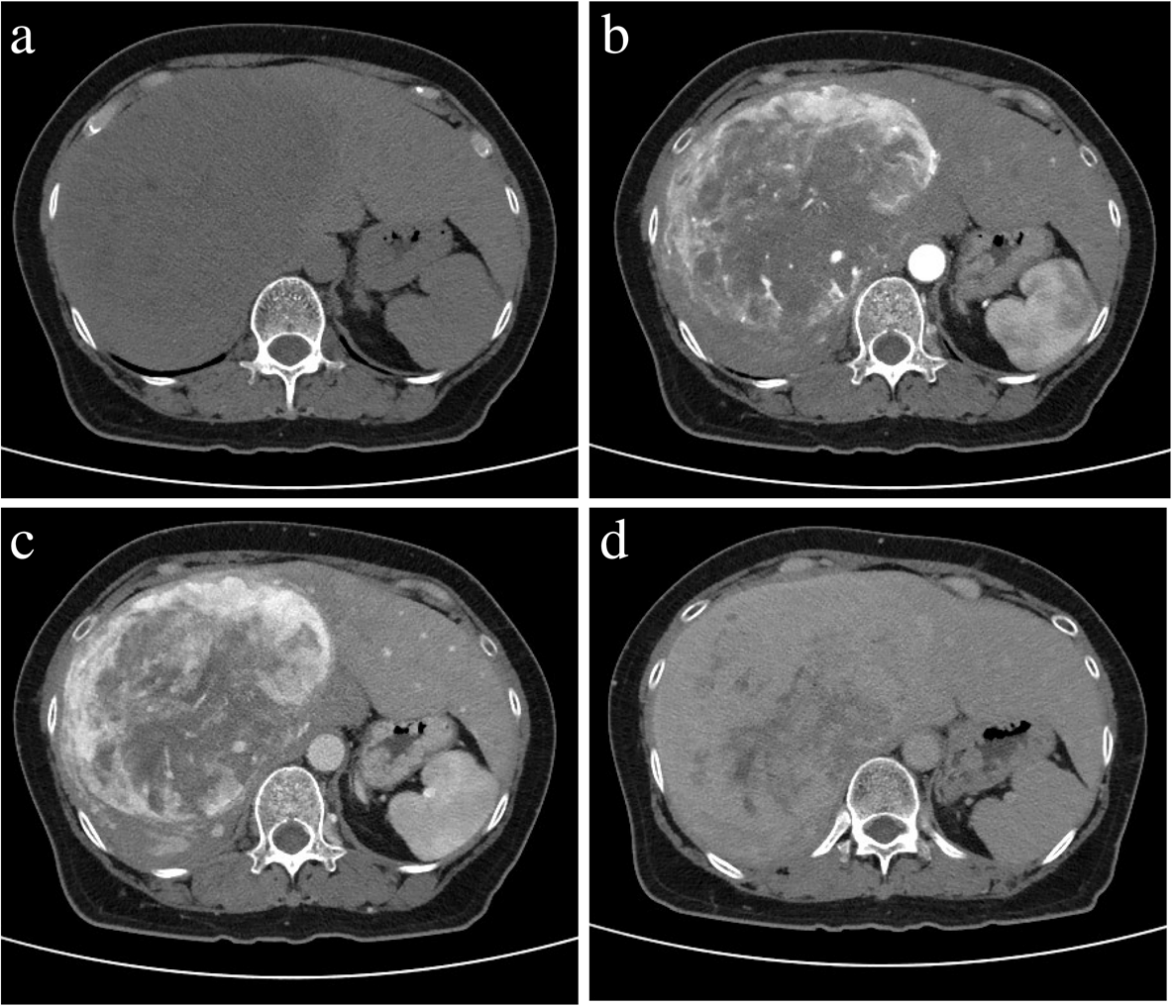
Contrast-enhanced abdominal computed tomography (CT). Plain CT shows a well-defined low-density mass occupying the right lobe (14.0 cm diameter) (a). Contrast-enhanced CT shows heterogeneous enhancement in the peripheral mass during the arterial phase (b). Enhancement is then centripetal and more pronounced during the portal phase (c) and finally progresses to persistent, less heterogeneous enhancement during the delayed phase (d)
